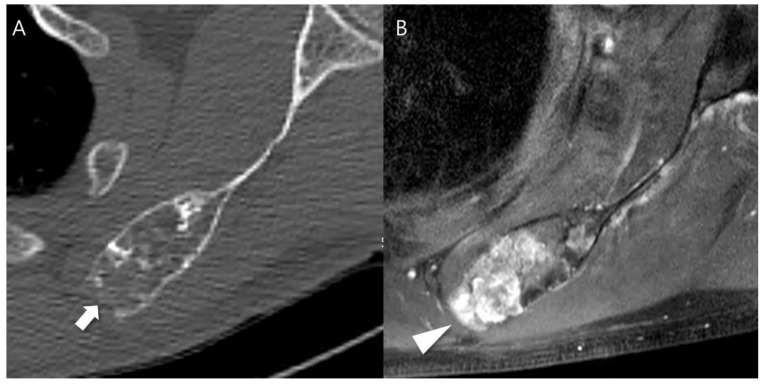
Cortical destruction with small extraosseous soft tissue extension in a patient with CS2 in the scapula. (A) An axial CT image shows a lobulated mass containing chondroid matrix mineralization and focal cortical destruction (arrow). (B) An axial T1-weighted fat-suppressed enhanced MRI shows a lobulated mass with extraosseous soft tissue extension (arrowhead).
